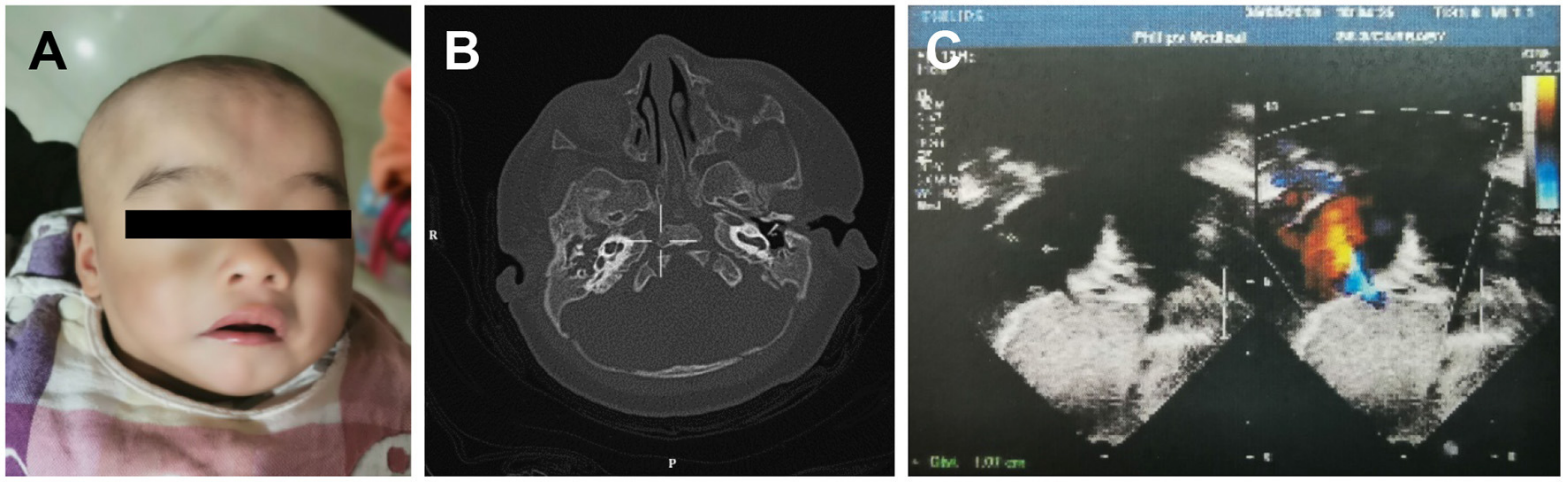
The developmental anomalies of the proband. (A) Patient shows facial dysmorphism. (B) Computerized tomography scan shows tympanosclerosis and blockage of the ear canals. (C) Echocardiogram showing a 1.1 cm atrial septal defect.
